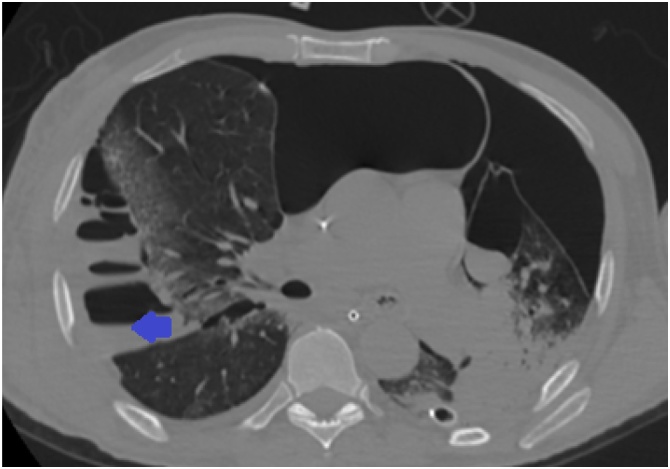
Thorax CT without contrast, bullectomy post-operative. The blue arrow indicates empyema and incarceration.
